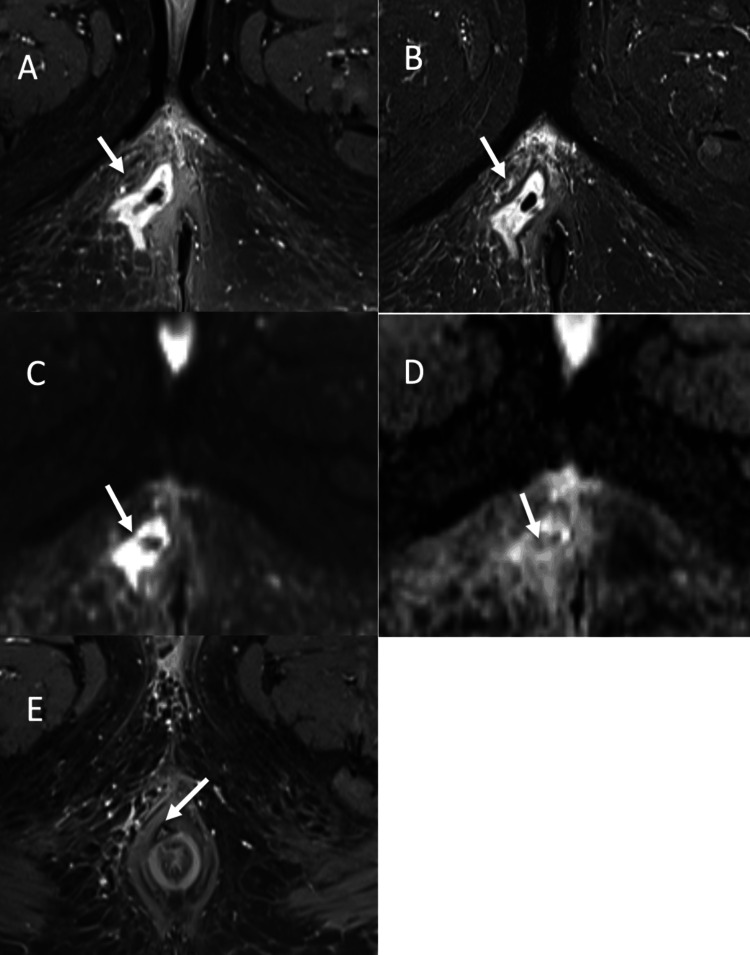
Perianal abscess in grade 4 trans-sphincteric fistula: (A-B) Axial post contrast fat suppressed T1-WI and axial STIR respectively, depicting a large heterogeneous collection with internal air foci in right perianal region showing peripheral enhancement on post contrast fat suppressed T1-WI (A) and hyperintense signal on T2 STIR (B). (C-D) Axial DWI and ADC respectively, depicting the collection showing hyperintense signal on DWI (C) with corresponding signal drop on ADC (D). (E) Axial post contrast fat supressed T1-WI showing a small trans-sphincteric fistula crossing the external sphincter at 11-o’clock position. T1-FS: T1 fat suppressed; STIR: short tau inversion recovery, DWI: diffusion weighted imaging; ADC: apparent diffusion coefficient
